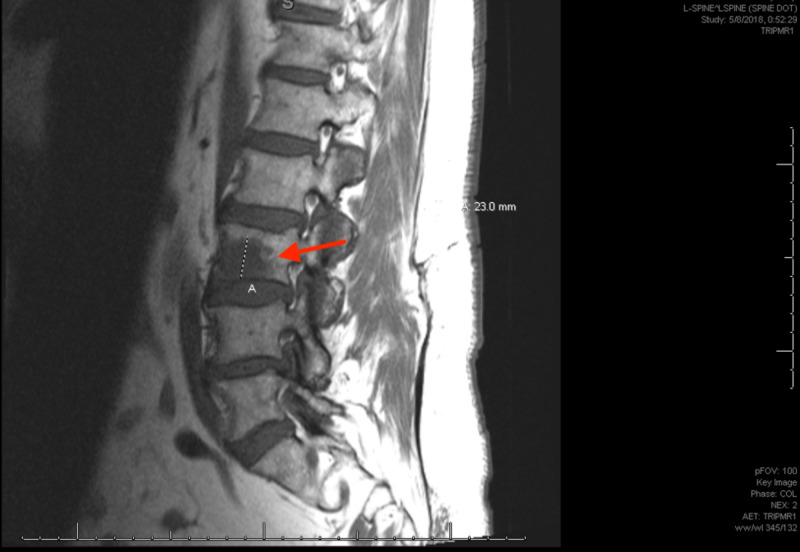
MRI of the spine revealing lytic lesions at L3.
